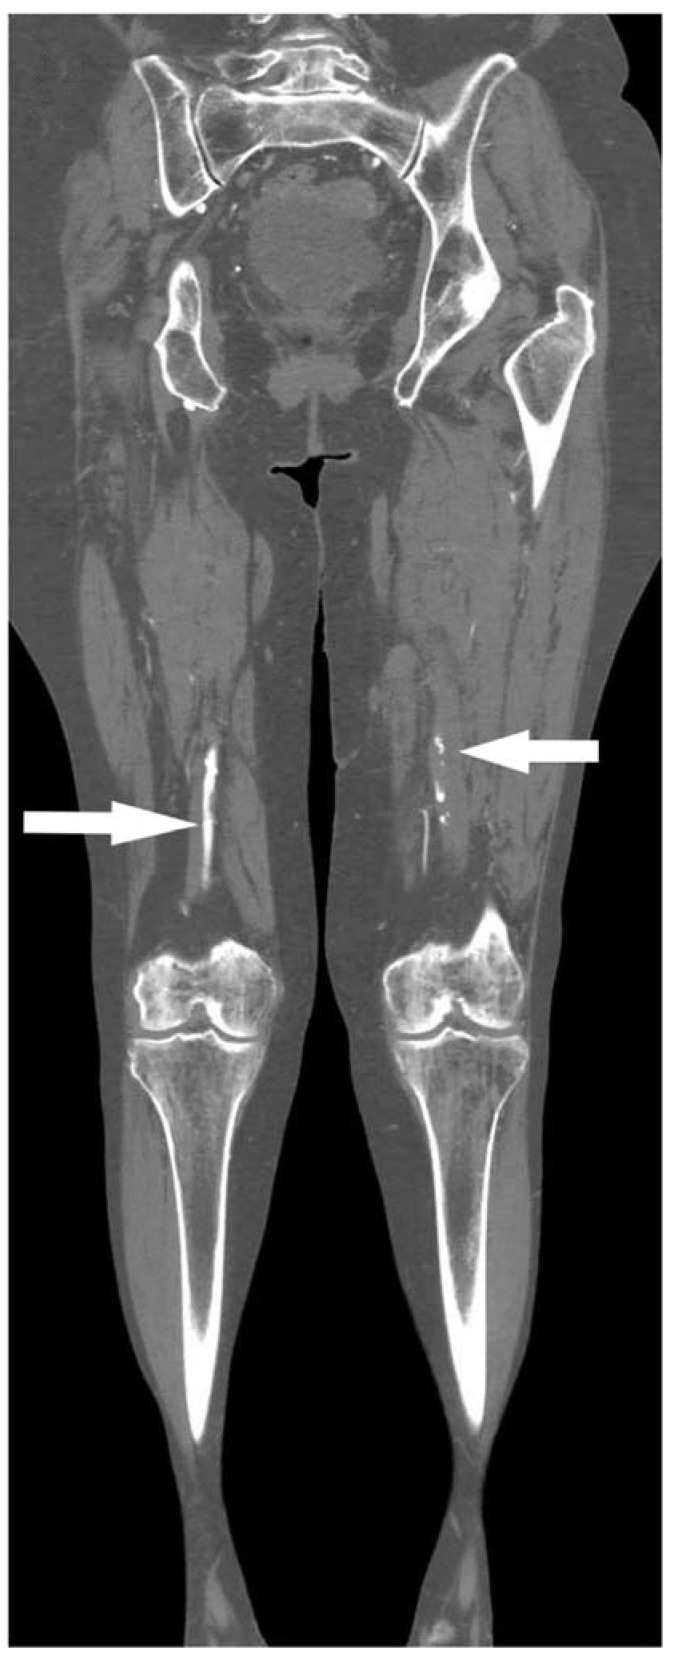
Coronal computed tomography angiography shows occlusion of the left common femoral artery (white arrows), the same patient as in Figure 2.
